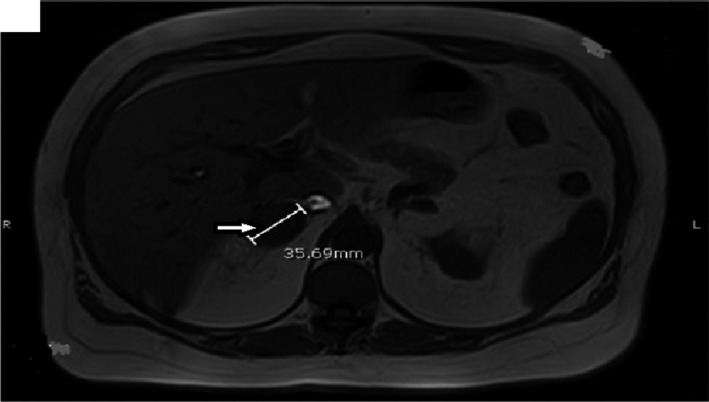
Magnetic resonance imaging (MRI) abdomen with and without contrast revealed a 3.6 cm right adrenal mass
